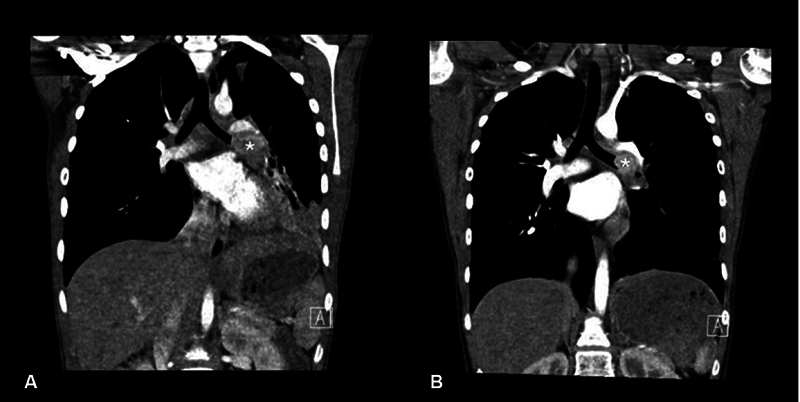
Coronal reformats of the thoracic contrast-enhanced diagnostic CT scans prior to treatment and following the second cycle of LuTATE therapy. The left bronchial carcinoid tumor is marked with an asterisk in each image. Prior to treatment (
